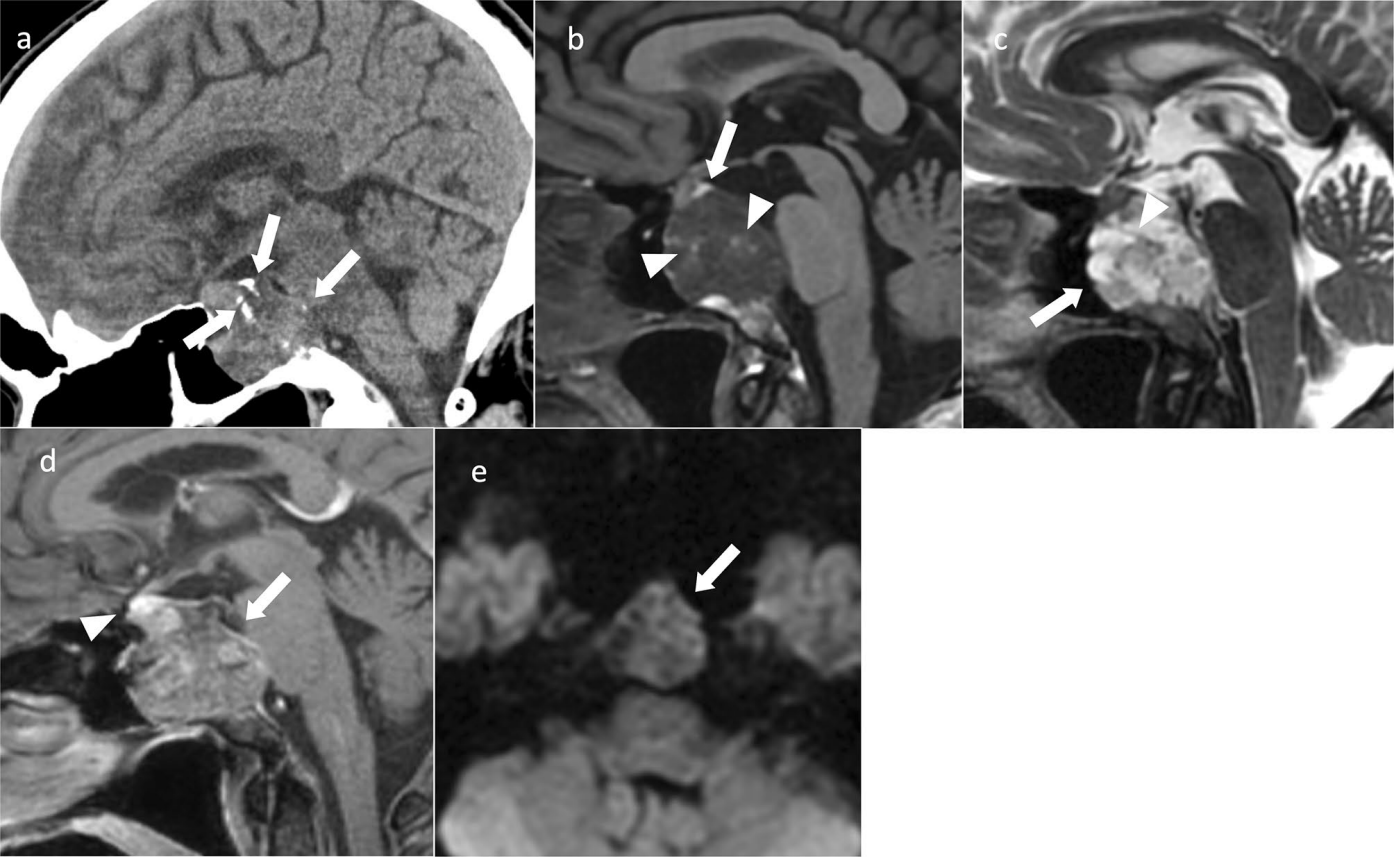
Clivus chordoma (conventional chordoma). An approximately 60-year-old male with polyopia. a Sagittal non-contrast computed tomography shows isodensity mass compared to white matter. Some hyperdensity area is observed suggesting sequestered fragments of the destroyed bone (arrows). b On sagittal T1WI, the mass shows hypointensity compared to white matter. Some small hyperintensity foci are observed in the mass (arrowhead). Pituitary gland is mildly pushed upward by the mass with normal hyperintensity in the posterior pituitary gland (arrow). c On sagittal T2WI, the mass shows marked hyperintensity (arrow) with hypointensity septations (arrowhead). d Sagittal contrast-enhanced T1WI shows moderate contrast enhancement in the mass (arrow), weaker than the pituitary contrast enhancement (arrowhead). e Diffusion-weighted imaging (DWI) shows isointensity compared to white matter in the mass (mean apparent diffusion coefficient = 1.4 × 10^–3^ mm^2^/s) (arrow)
